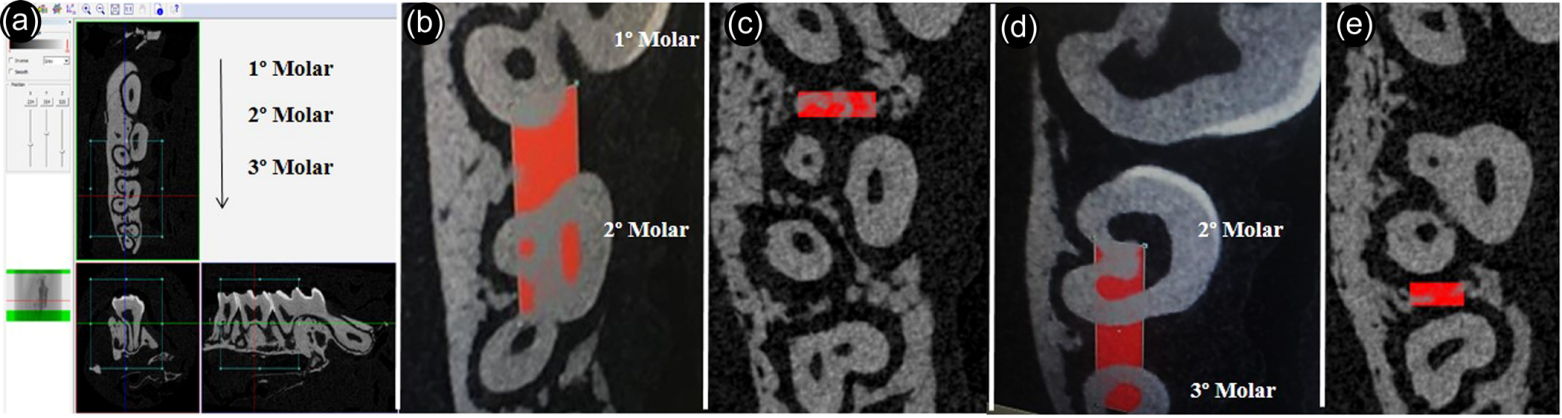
Micro‐CT analysis. (a) Measurement in the CT Analyzer software in the interproximal region for volumetric analysis by means of a sagittal image of the maxillary second molar. (b) Delimitation of the mesial region of interest (ROI) mesial interproximal region. (c) Measurement of mesial region 4. Delimitation of the ROI distal interproximal region. (d) Measurement of the distal region.
